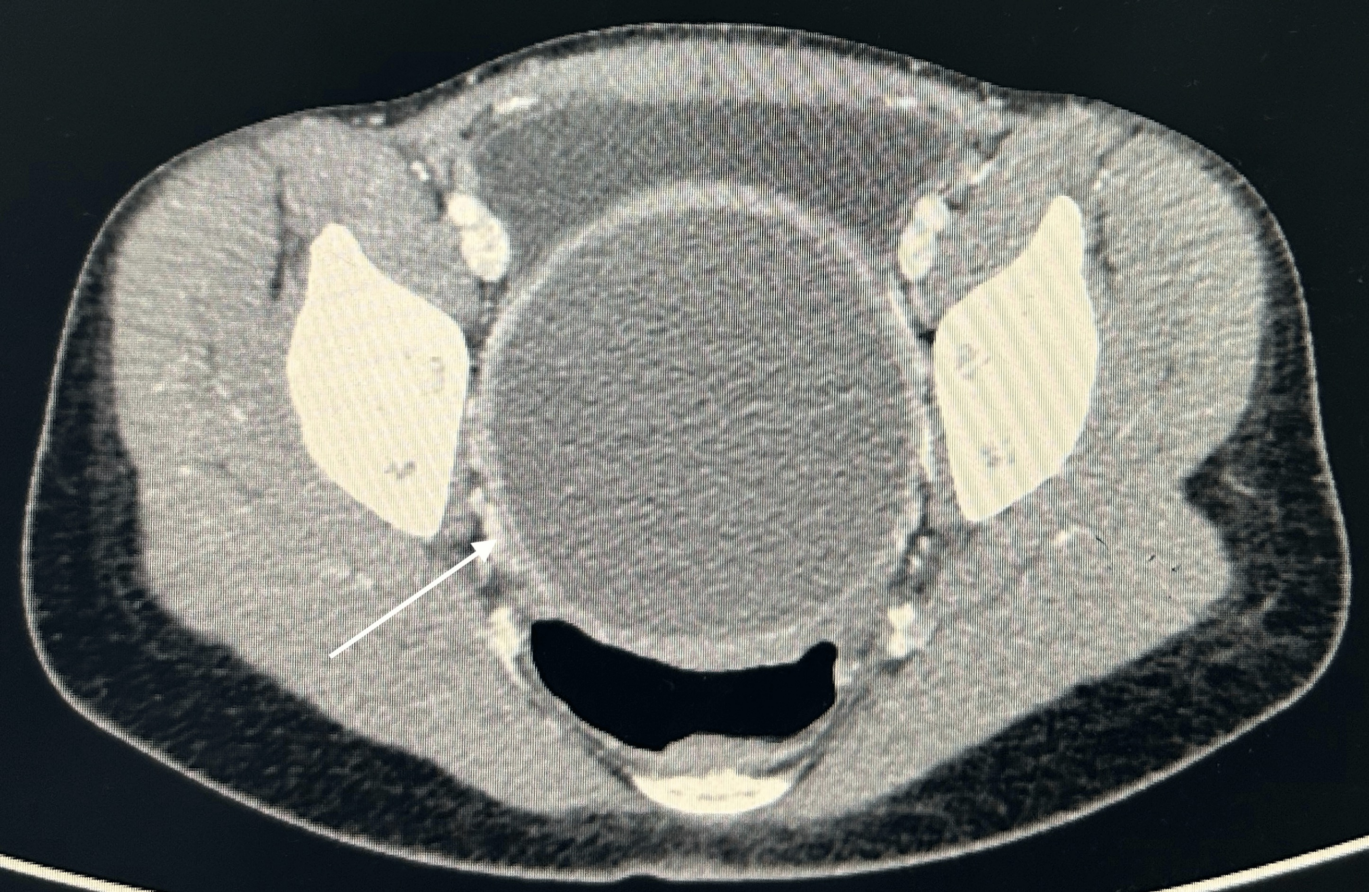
CT axial view with a fluid-filled uterine cavity CT: computed tomography
